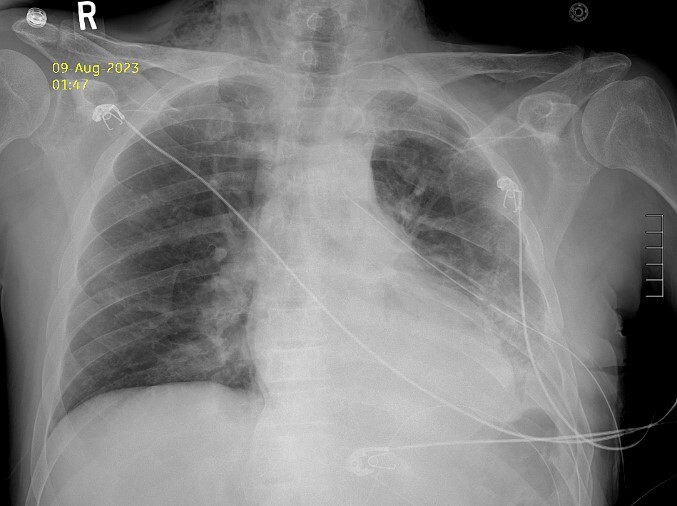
Postoperative Day 2 chest X-ray showing reduced diaphragmatic hernia and resolving pleural effusion.
